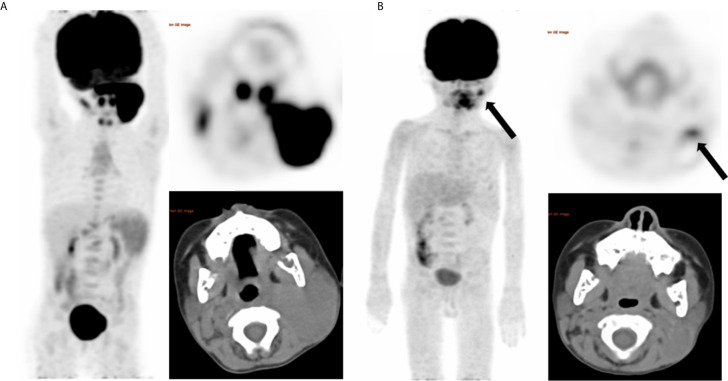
A representative case of a 4-year-old female with stage IIIB BL and nodal disease. Baseline maximum intensity projection (MIP) (A) showing hypermetabolic lesion in cervical nodes. End of treatment PET/CT (B) showing moderate 18F-FDG uptake residual lesion in the cervical node (SUVmax = 4.1), considering Deauville score of 4 and a partial response. And the residual lesion dissection was performed, proving no viable lymphoma cell. After a follow up time of 62 months, this patient did not develop relapse and was alive.
